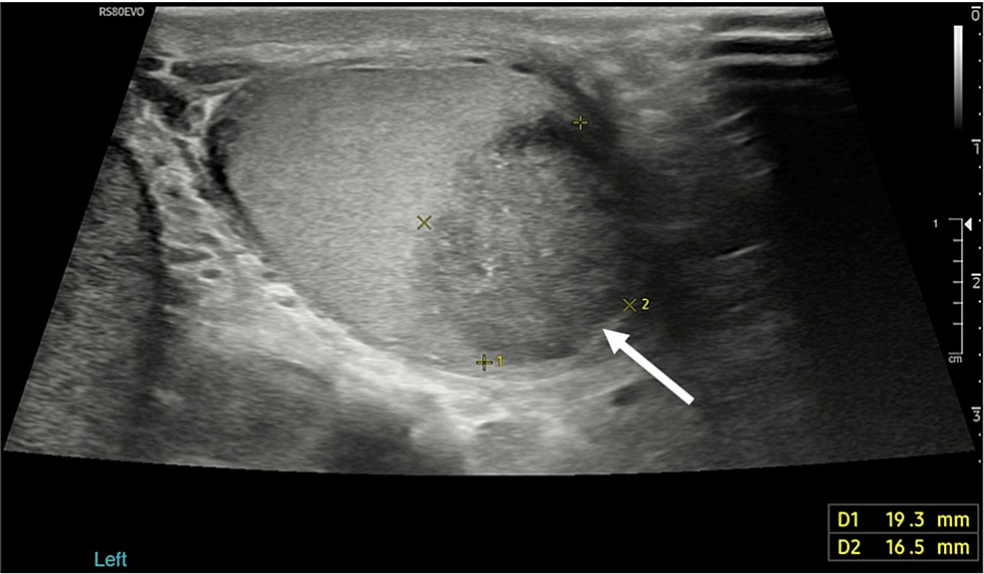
Ultrasound testes revealed a 19 mm x 17 mm left upper pole testicular mass with hyperechoic flecks
